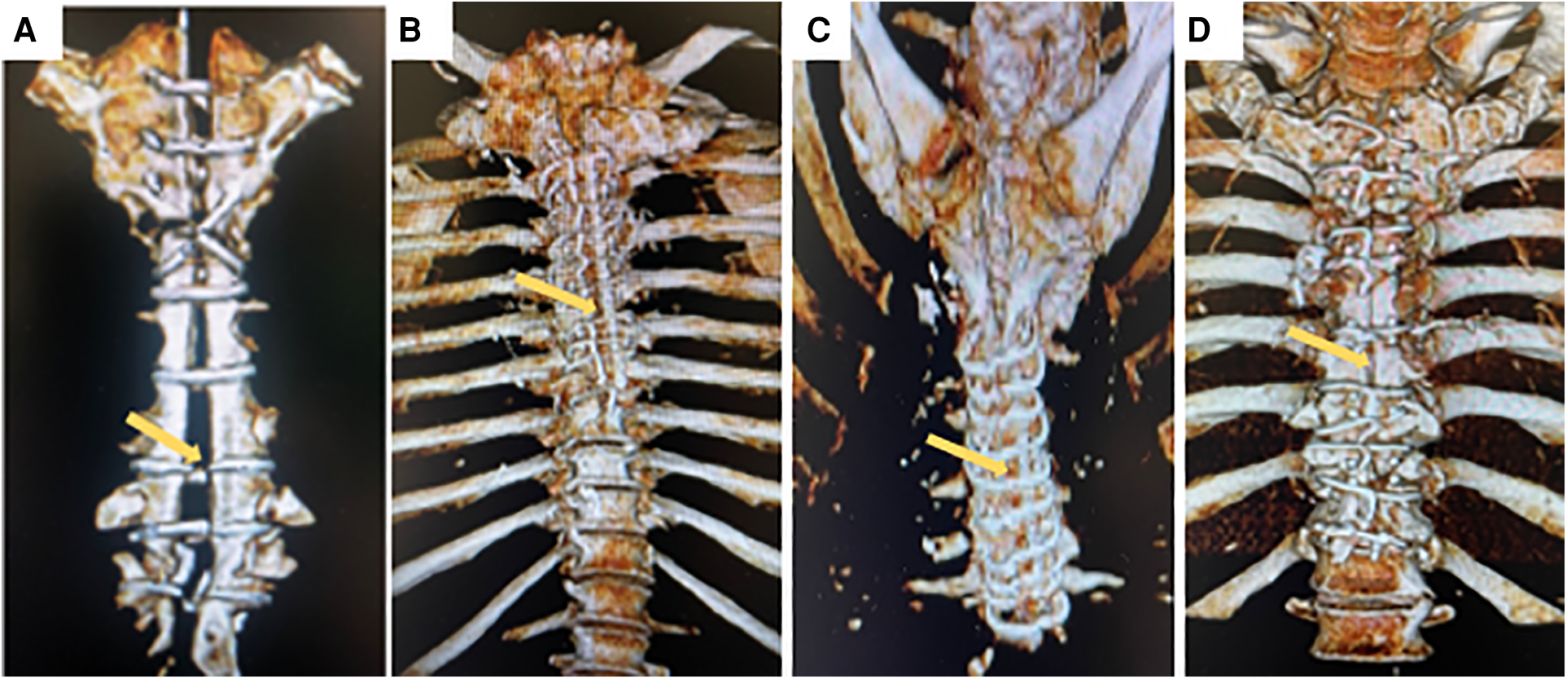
Representative three-dimensional computed tomography scans for patient with deep sternal infection with sternal dehiscence and mediastinitis (yellow arrow) (A) and same patient after chest wall reconstruction with complete bone healing (yellow arrow) (B). Patient with complete sternal healing after chest reconstruction (C), Patient with incomplete sternal healing after conventional sternal closure (D).
